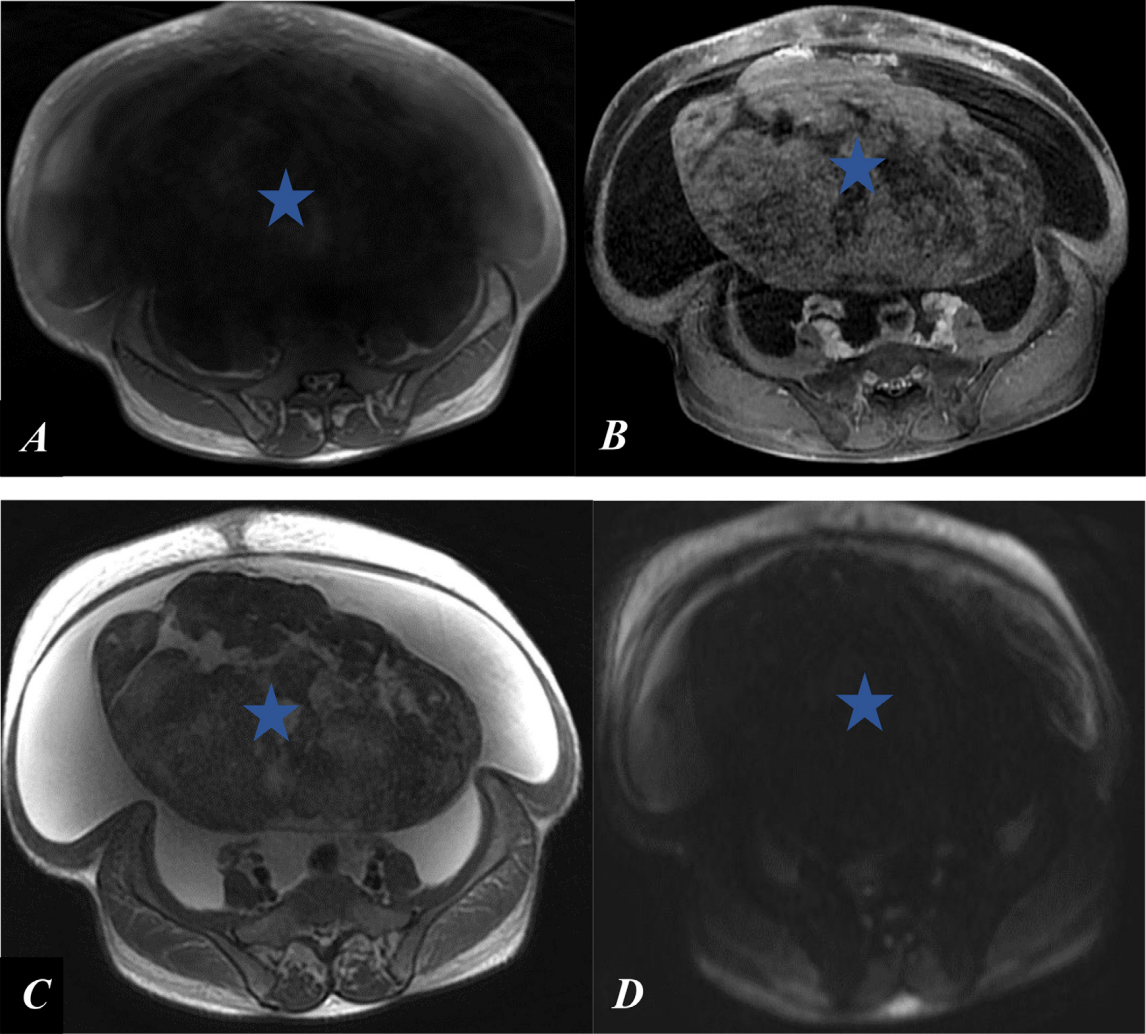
T1-weighted MRI sequence without contrast (A), contrast-enhanced T1-weighted sequence (B), diffusion-weighted sequence (D), and T2-weighted sequence (C) demonstrating a right ovarian mass, hypointense on T1 and T2, nonrestrictive on diffusion, and moderately enhanced after gadolinium administration (blue star).
